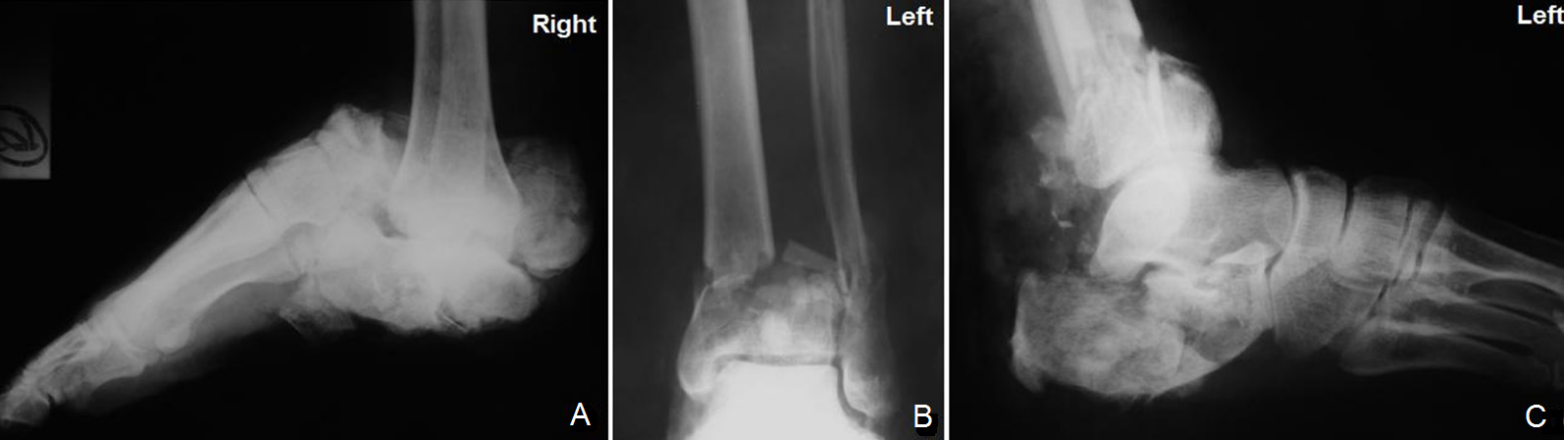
A lateral radiograph of the right foot (A) and anteroposterior (B) and lateral (C) radiographs of the left lower extremity at the time of injury.
